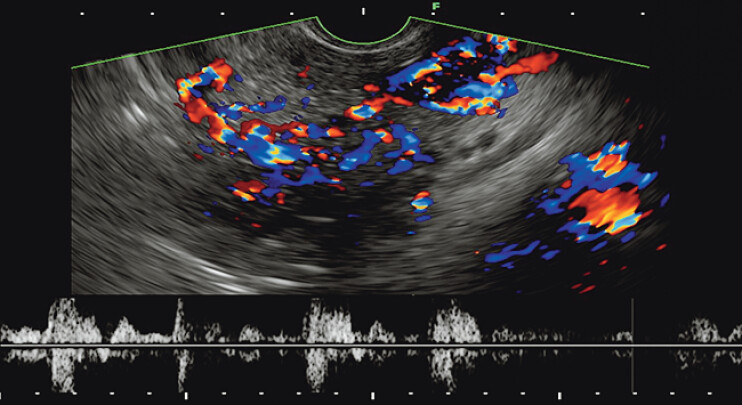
The application of color Doppler imaging contributed to the identification of P-AVM. Color Doppler imaging revealed tortuous and dilated blood vessels. Spectral Doppler analysis demonstrated arterial pulsatile flow characteristics.
